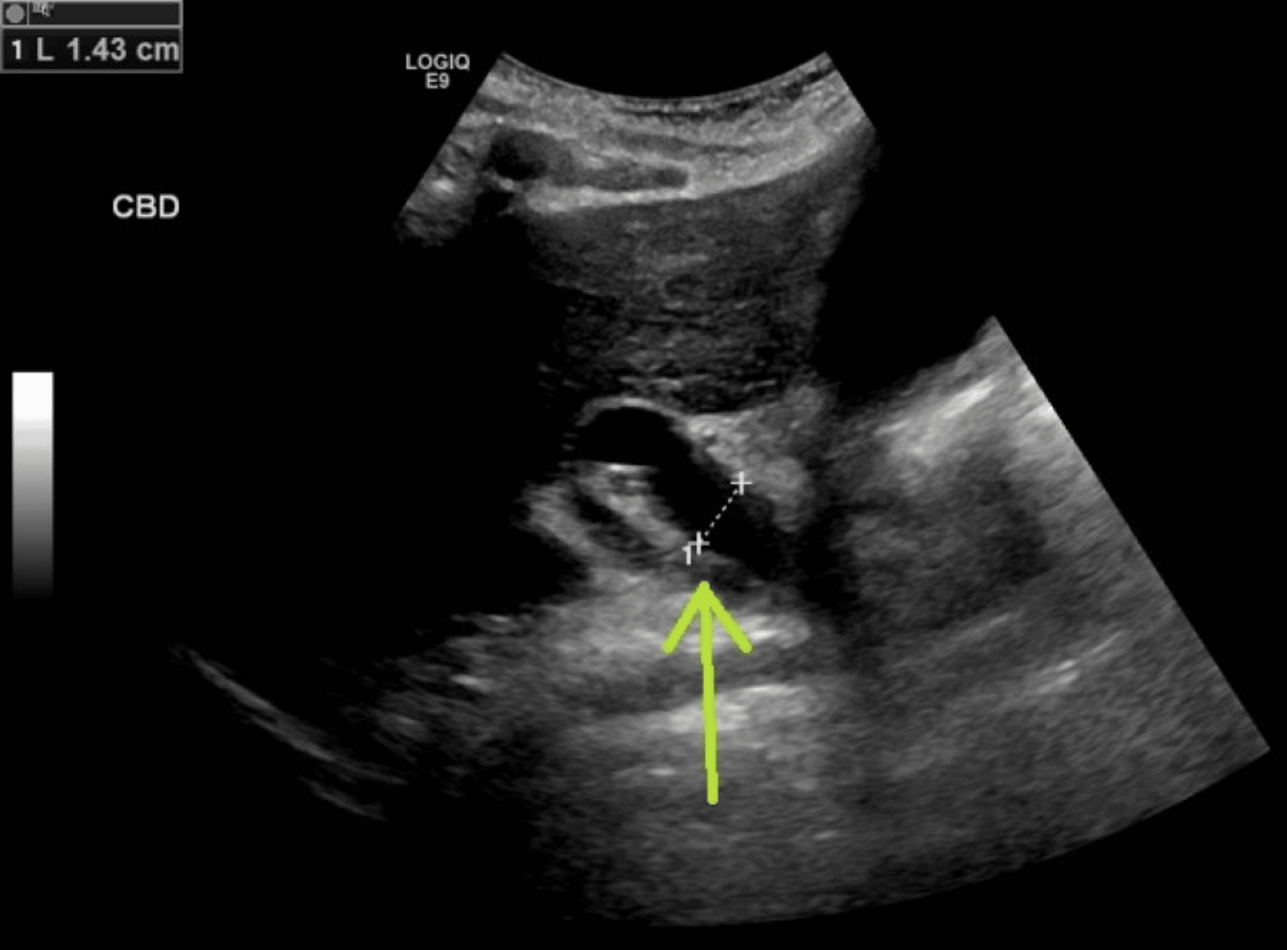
Right upper quadrant ultrasound demonstrating a dilated CBD.
